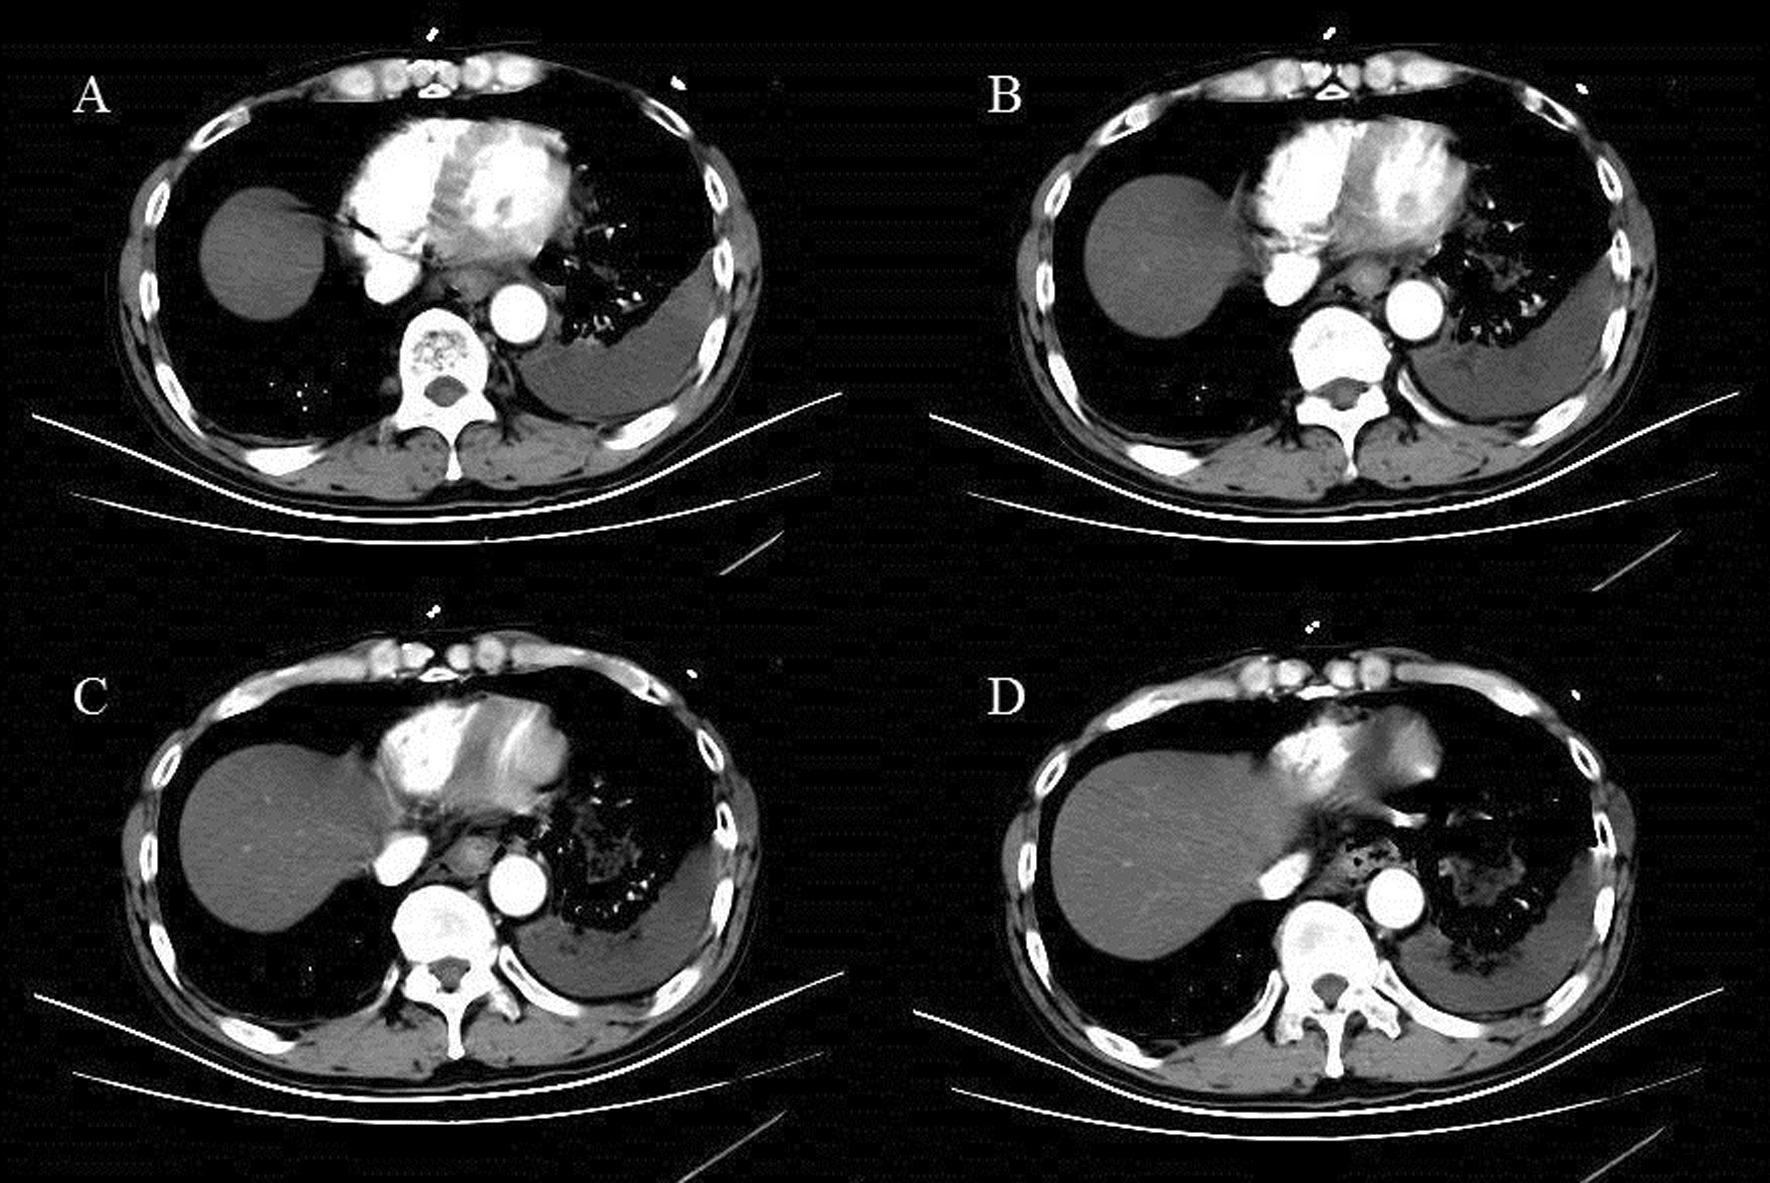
CT showing pneumomediastinum with air tracking laterally towards the left pleural space, and a large left pleural effusion
